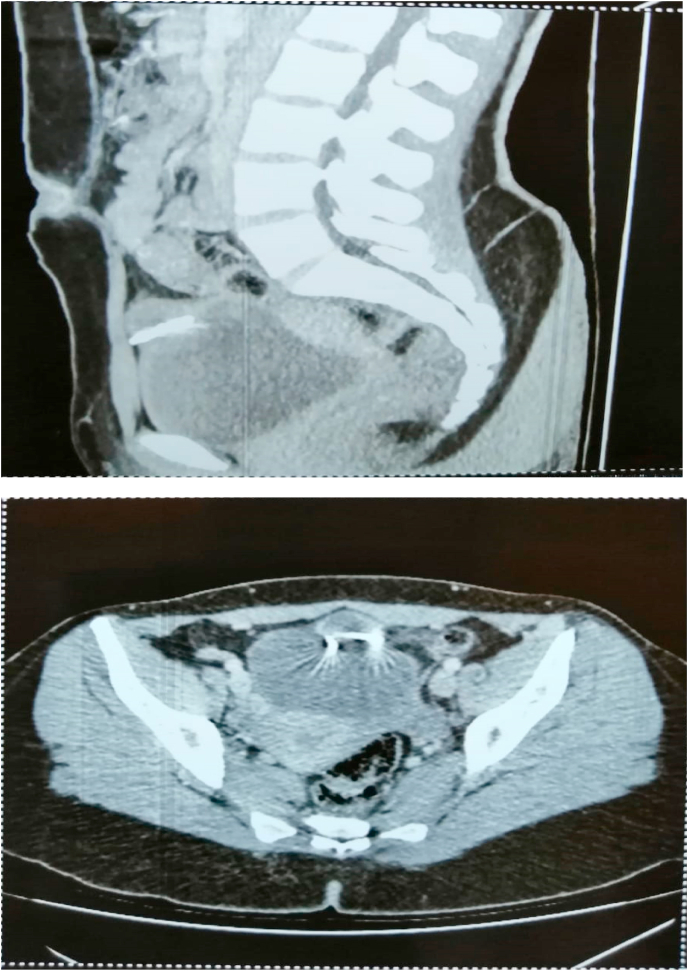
Abdomino-pelvic CT showed on a sagittal section and an axial section: an anteverted uterus with an IUD above the bladder associated with a collection came into contact with the anterior wall of the bladder with conservation of the separation line.
